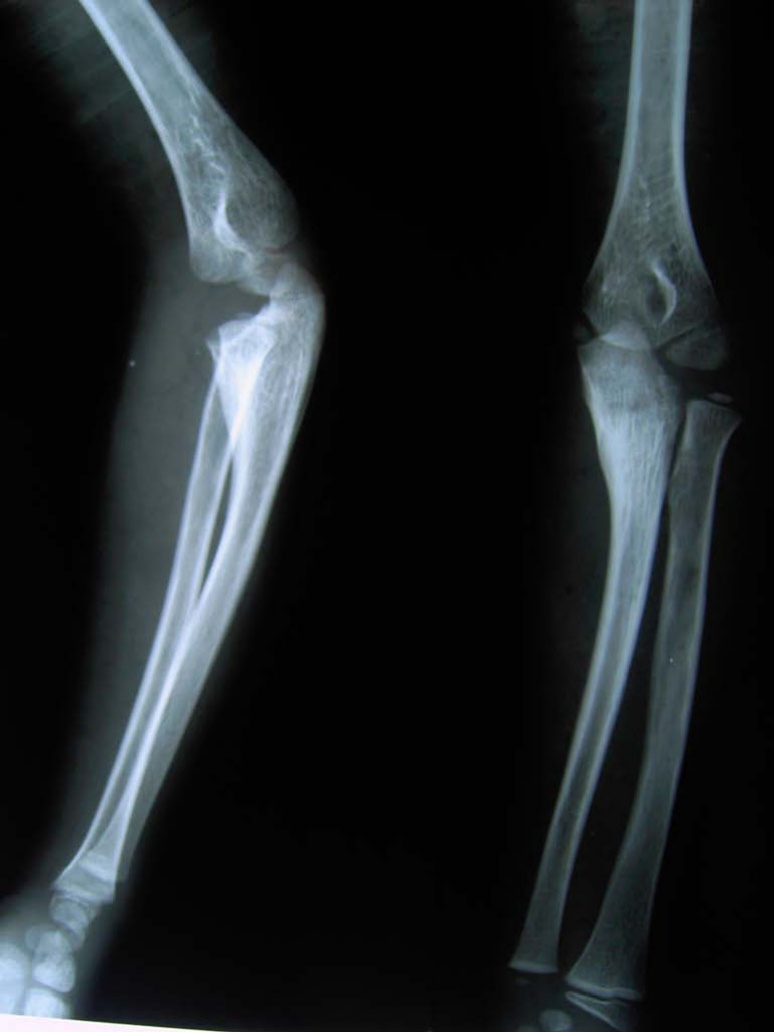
Preoperative radiograph showing radial head dislocation (Patient 2).
